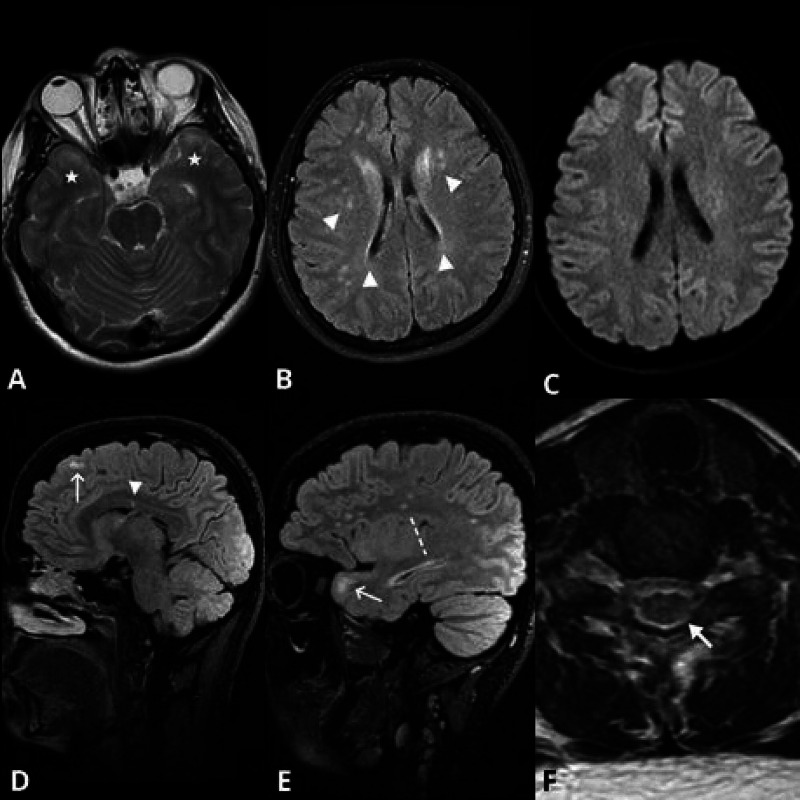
On axial T2 weighted MR and Sagittal 3D CUBE fluid-attenuated inversion recovery (FLAIR) images On axial T2 weighted MR image, increased signal intensity is seen at bilateral anterior temporal subcortical white matter (asterisks, image A). Image B demonstrates periventricular plaques and numerous high signal intensity foci at corona radiata (arrow heads). There is no diffusion restriction appreciated in our case (image C). Sagittal 3D CUBE FLAIR images show corpus callosum lesion (arrow head) and juxtacortical lesions (thin arrows, images D and E). Also, frontoparietal white matter lesions (dashed line) are well demonstrated on image E. In addition to cranial images, on axial T2 weighted image of the spine (image F), there is an increased signal focus (thick arrow) detected at left half of posterior spinal cord.
